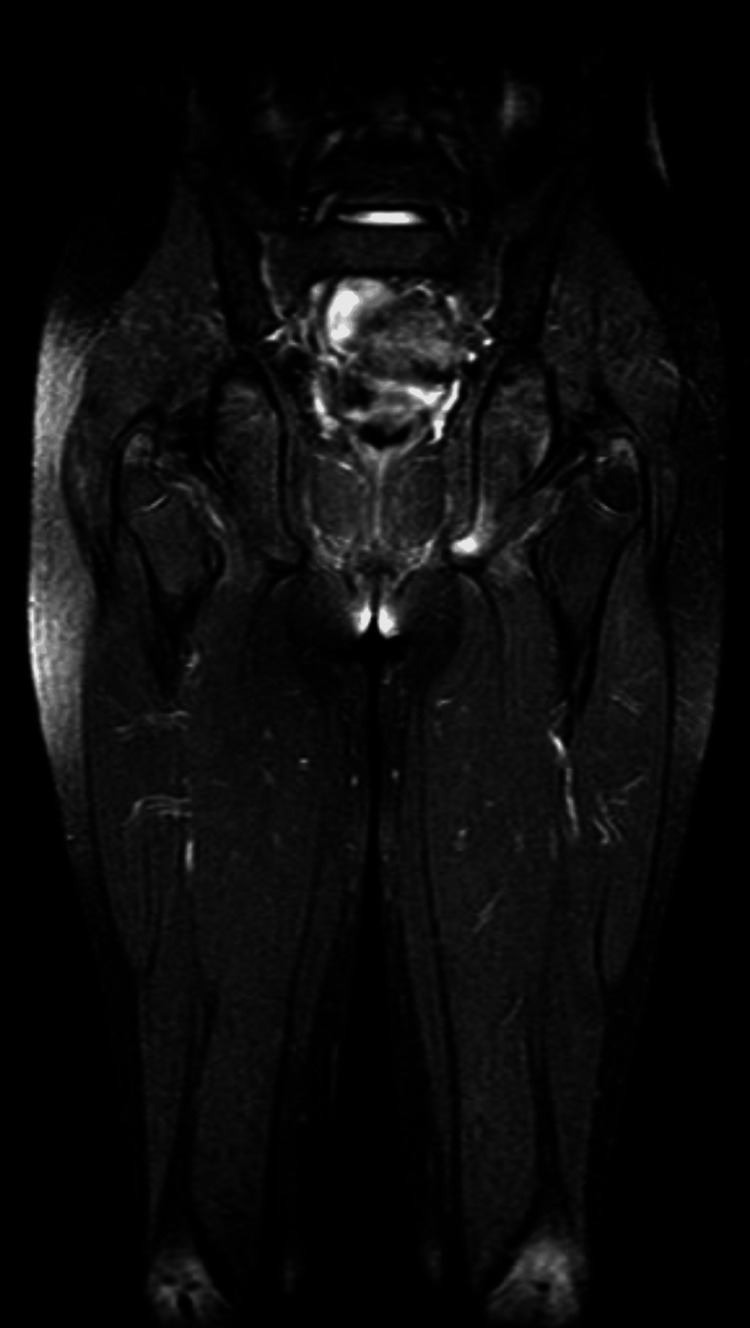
Coronal T2-weighted fat-suppressed MRI of the pelvis and proximal thighs showing diffusely hyperintense muscles, indicative of inflammatory myopathy.
